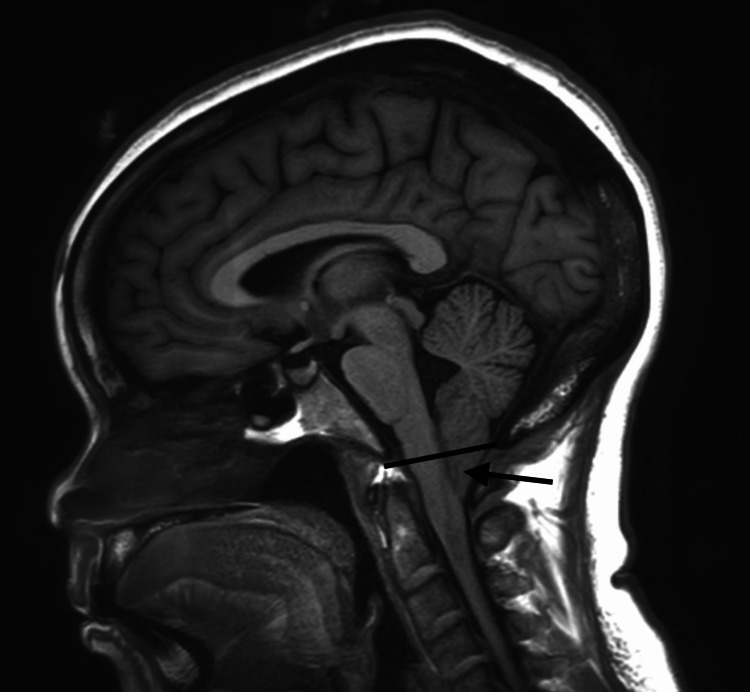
Sagittal T1-Weighted MRI brain showing 15.5 mm tonsillar protrusion (arrow)
